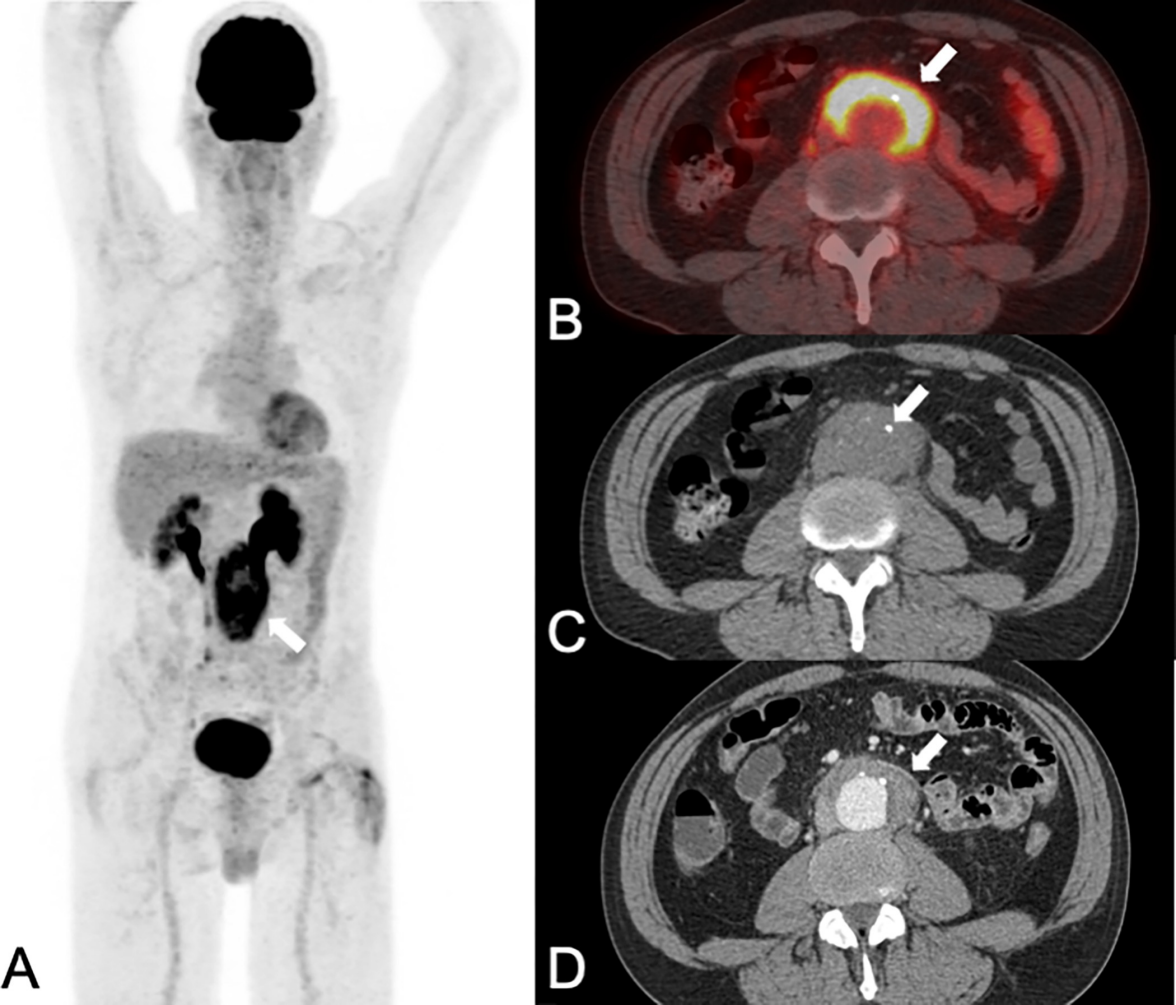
A 58-year-old man with an immunoglobulin G4-associated inflammatory abdominal aortic aneurysm (Ormond disease), initially presented with back pain and fever. PET/CT (A: maximum intensity projection of PET; B: fused PET/CT images; C: native CT images) and contrast-enhanced CT (CE-CT: D) showed a 64 mm large abdominal aortic aneurysm (white arrows in A-D) with intense FDG uptake (SUVmax 9.0 in A and B) in the thickened (12 mm) aortic wall (white arrows in B-D) with prominent dorsal sparing. Using PET/CT images alone one reader correctly diagnosed an inflammatory aneurysm, while the other one falsely diagnosed an infectious/mycotic aneurysm. With CE-CT and with combined imaging both readers correctly diagnosed an inflammatory aneurysm. The patient was subsequently treated with percutaneous endovascular abdominal aortic aneurysm repair and steroid therapy. The latest follow-up imaging 2.5 years after the initial diagnosis (not shown), showed a smaller (42 mm) aneurysm with less FDG uptake (SUVmax 4.1); and the patient had no symptoms.
